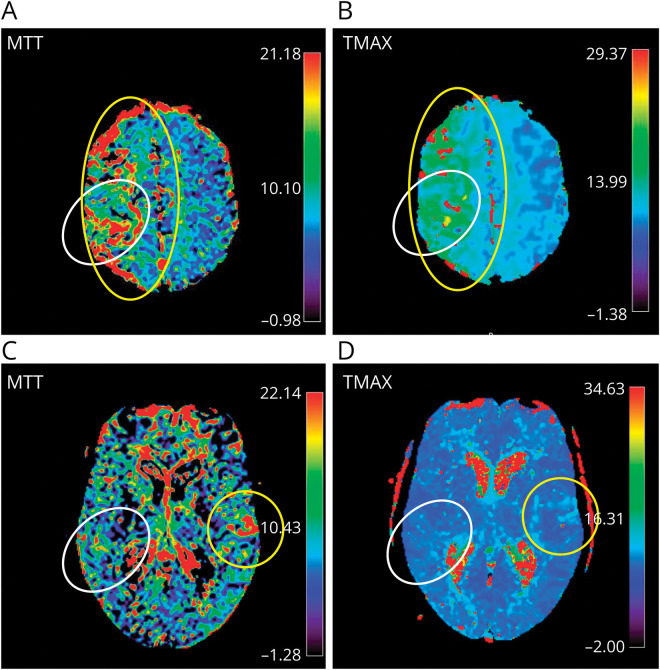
Assessment of Focal Hypoperfusion Within and Outside the Expected TIA Territory on Baseline Perfusion-Weighted Imaging MRI (A, B) Focal hypoperfusion within the expected TIA territory: PWI of a 71-year-old woman after 2 episodes of left facio-brachio-crural hemiparesis for 20 minutes with an expected TIA territory in the right middle cerebral artery territory (white circle). Focal hypoperfusion (yellow circle for perfusion delay) lies within the expected TIA territory in the right hemisphere and corresponds to prolonged MTT (up to 21 seconds, A) and prolonged Tmax (up to 15 seconds, B). TIA etiology was a symptomatic extracranial stenosis of the internal carotid artery. (C, D) Focal hypoperfusion outside the expected TIA territory: PWI of an 82-year-old woman after an episode of left-sided facial paresis for 20 minutes with the expected TIA area in the right hemisphere (white circle). There was no focal hypoperfusion within the expected TIA territory, but rather outside it (yellow circle), in the left opercular area, corresponding to slight prolongation of MTT (up to 20 seconds, C) and Tmax (up to 12 seconds, D). A specific TIA etiology could not be determined. MTT = mean transit time; PWI = perfusion-weighted imaging; Tmax = time-to-maximum.
